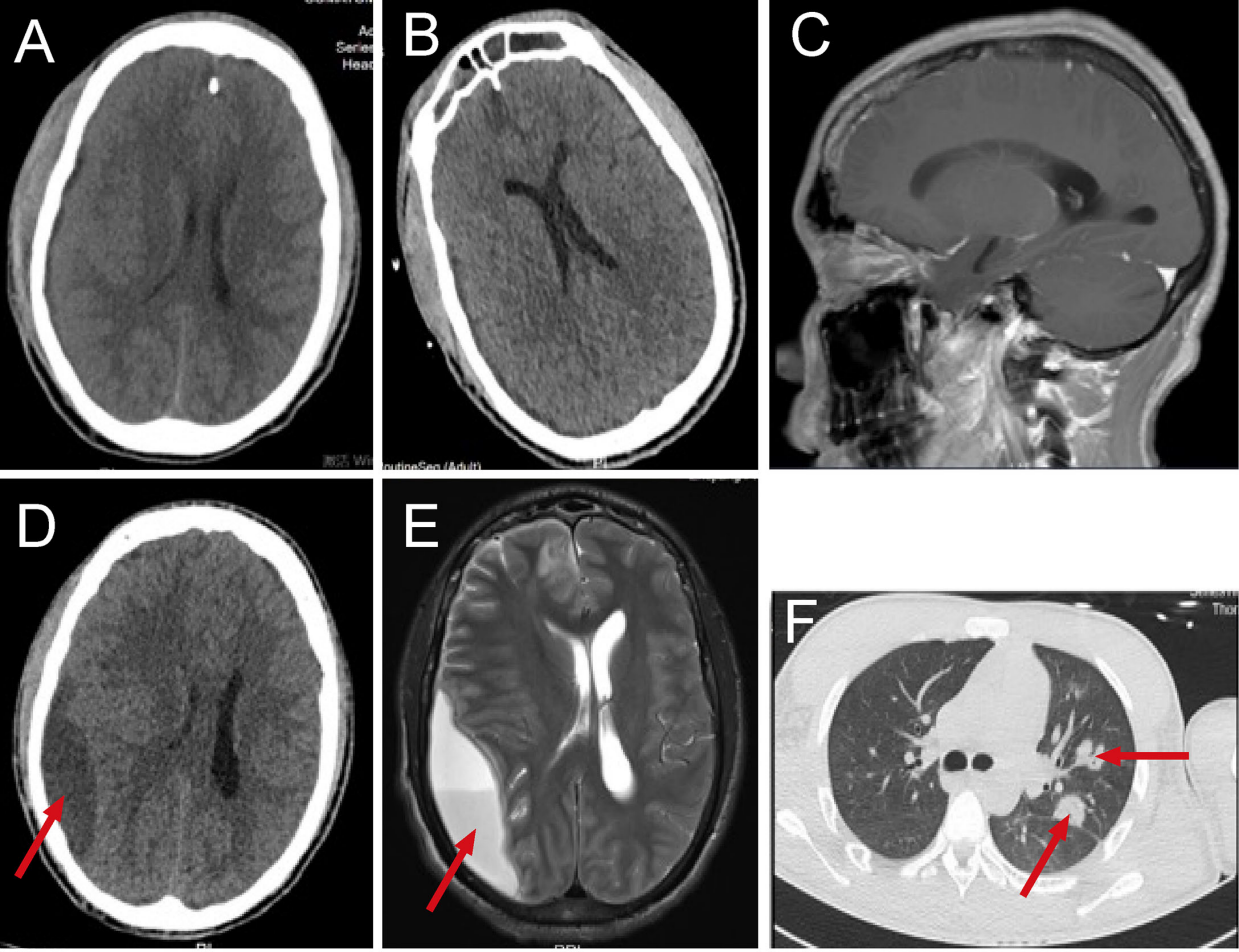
The dynamic imaging examination in the pre-operative period. Head computed tomography(CT) scan at admission (A), head CT on the 7th day of hospitalization (B), the patient’s dental status on magnetic resonance imaging (MRI) (C), head CT on the 18th day of hospitalization (D), head MRI on the 21th day of hospitalization (E), lung CT on the 18th day of hospitalization (F).
